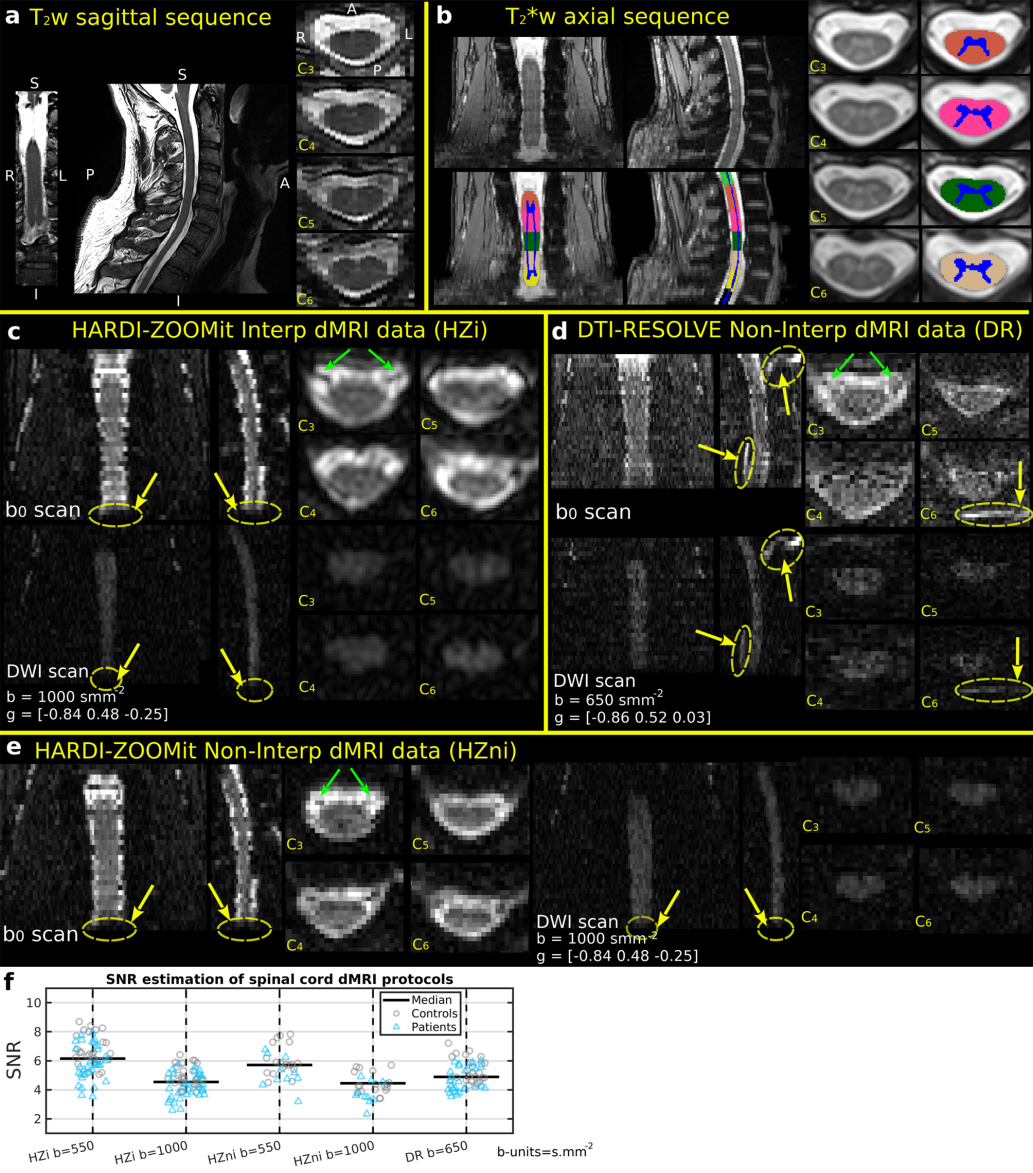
Raw anatomical MRI data, white and gray matter segmentation, and vertebral level labeling in a single-subject (a–e). Coronal (left), sagittal (middle) and axial (right) images with corresponding cervical (C) vertebral levels are shown; anatomical orientation: *S* superior, *I* inferior, *R* right, *L* left, *A* anterior, *P* posterior, as included in (a); anatomical plane order and direction orientation is the same in all other panels. (a), $$\hbox {T}_2\hbox {w}$$ sagittal sequence (b), $$\hbox {T}_2^*\hbox {w}$$ axial sequence with WM/GM segmentation and vertebral level labeling overlay (c). Diffusion non-weighted (i.e. $$\hbox {b}_0$$ scan in the first row of images) and weighted (i.e. DWI scan in the second row of images) images for the HARDI-ZOOMit protocol with Fourier domain interpolation during image reconstruction. Variable b (i.e. b-value) represents a magnitude of applied gradient waveform in the direction of a gradient vector g. Yellow arrows indicate areas of signal loss, green arrows the nerve roots in $$\hbox {b}_0$$ scan. (d) $$\hbox {b}_0$$ and DWI scans for the non-interpolated DTI-RESOLVE protocol. Order of $$\hbox {b}_0$$ and DWI scans and diffusion variable description same as in (c). Yellow arrows indicate locations of ghost artifacts, green arrows the nerve roots in $$\hbox {b}_0$$ scan. (e) $$\hbox {b}_0$$ (left) and DWI (right) scans for the non-interpolated HARDI-ZOOMit protocol. Diffusion variable description same as in (c). Yellow arrow indicate areas of signal loss, green arrows the nerve roots in $$\hbox {b}_0$$ scan. (f) Estimation of signal-to-noise ratio (SNR) in spinal cord DWI scans of investigated protocols.
